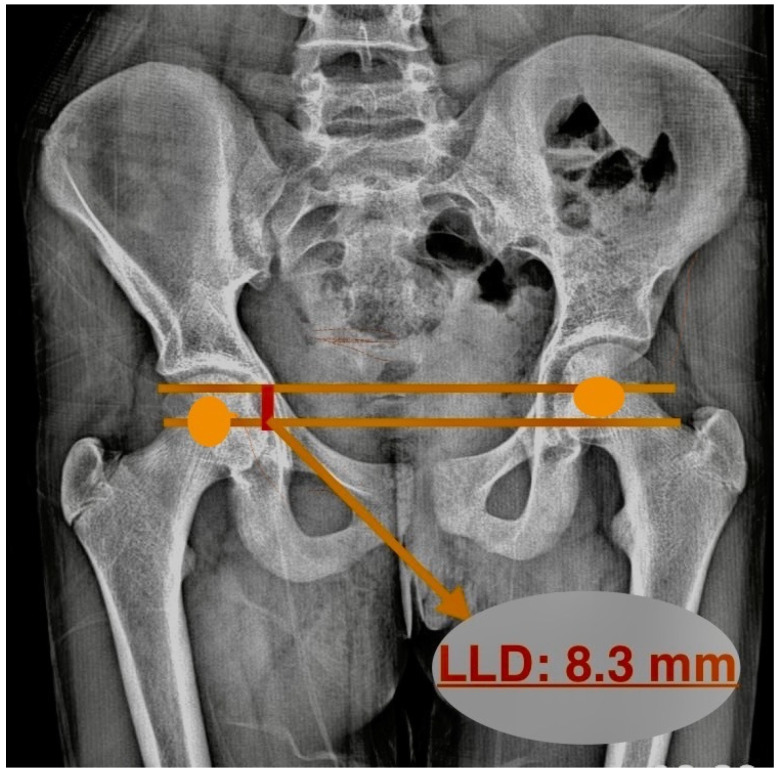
Measuring leg length discrepancy on radiograph.
